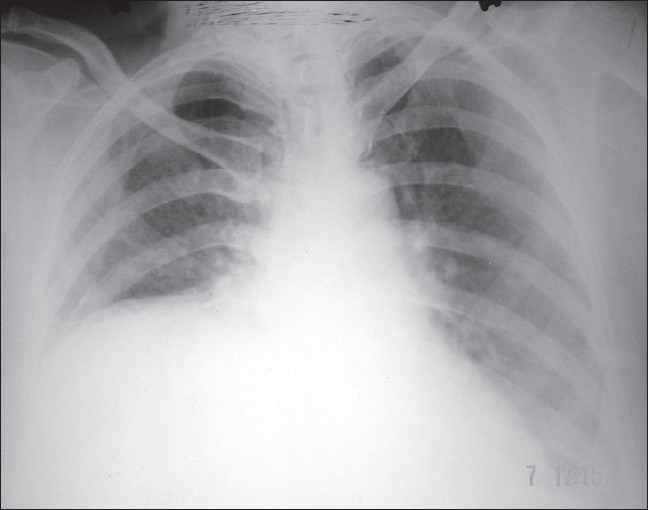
Chest X-ray posteroanterior view showing eventration of right dome of diaphragm
